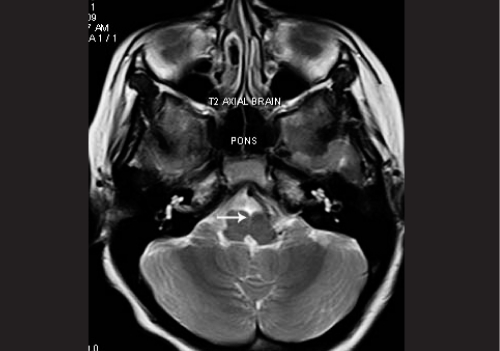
MRI brain of case 2, T2 axial, arrow denotes pontine cleft
